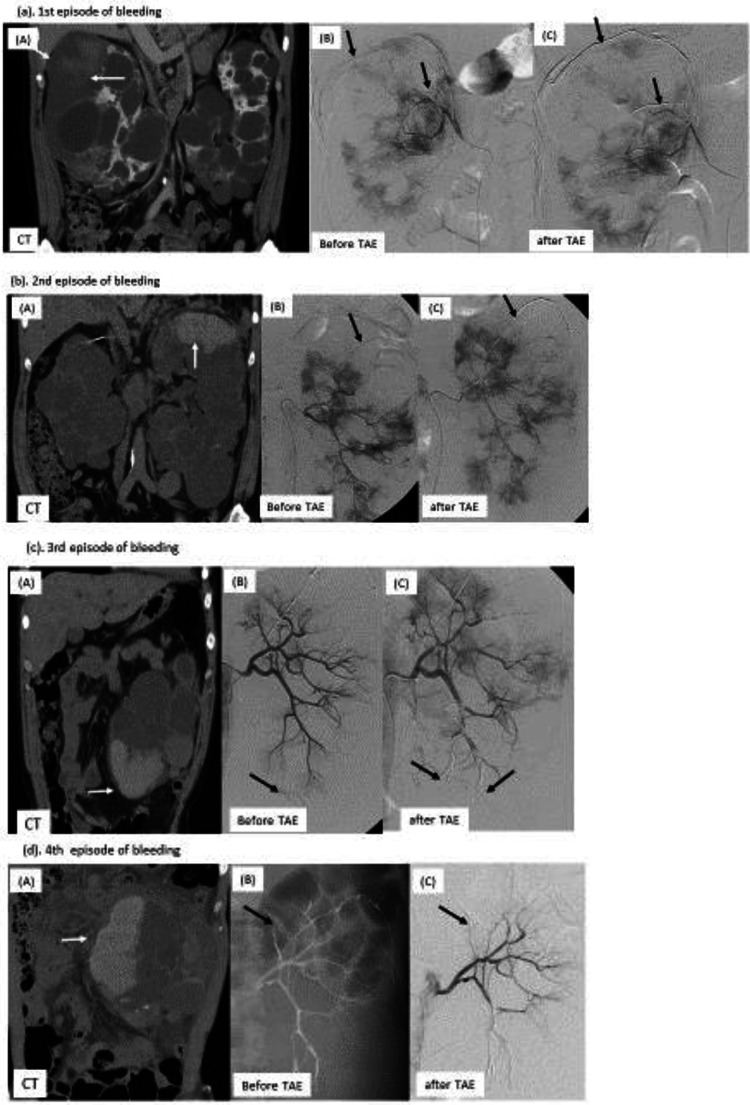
a First bleeding episode A: Coronal computed tomography (CT) showed bleeding into the right subcapsular space (arrow). B: Right renal angiogram showed bleeding from the right capsular artery branch (arrow). C: Renal transarterial embolization (TAE) with four microcoils (arrow) was performed on the right capsular artery branch. b: Second bleeding episode A: CT showed bleeding into the subcapsular space of the upper pole of the left kidney. B: Left renal angiogram showed bleeding from the left superior capsular artery branch (arrow). C: Renal TAE with four microcoils (arrow) was performed on the left superior capsular artery branch (arrow).c: Third bleeding episode A: CT showed bleeding into the subcapsular space of the inferior pole of the left kidney (arrow). B: Left renal angiogram showed bleeding from the inferior pole branch of the left renal artery (arrow). C: Renal TAE with four microcoils (arrow) was performed on the inferior pole branch of the left renal artery. d: Fourth bleeding episode A: CT showed bleeding into the subcapsular space in the medial part of the upper pole of the left kidney (arrow). B: Left renal angiogram showed bleeding from the medial part branch of the upper pole of the left kidney. C: Renal TAE with four microcoils (arrow) was performed on the medial part branch of the upper pole of the left kidney
